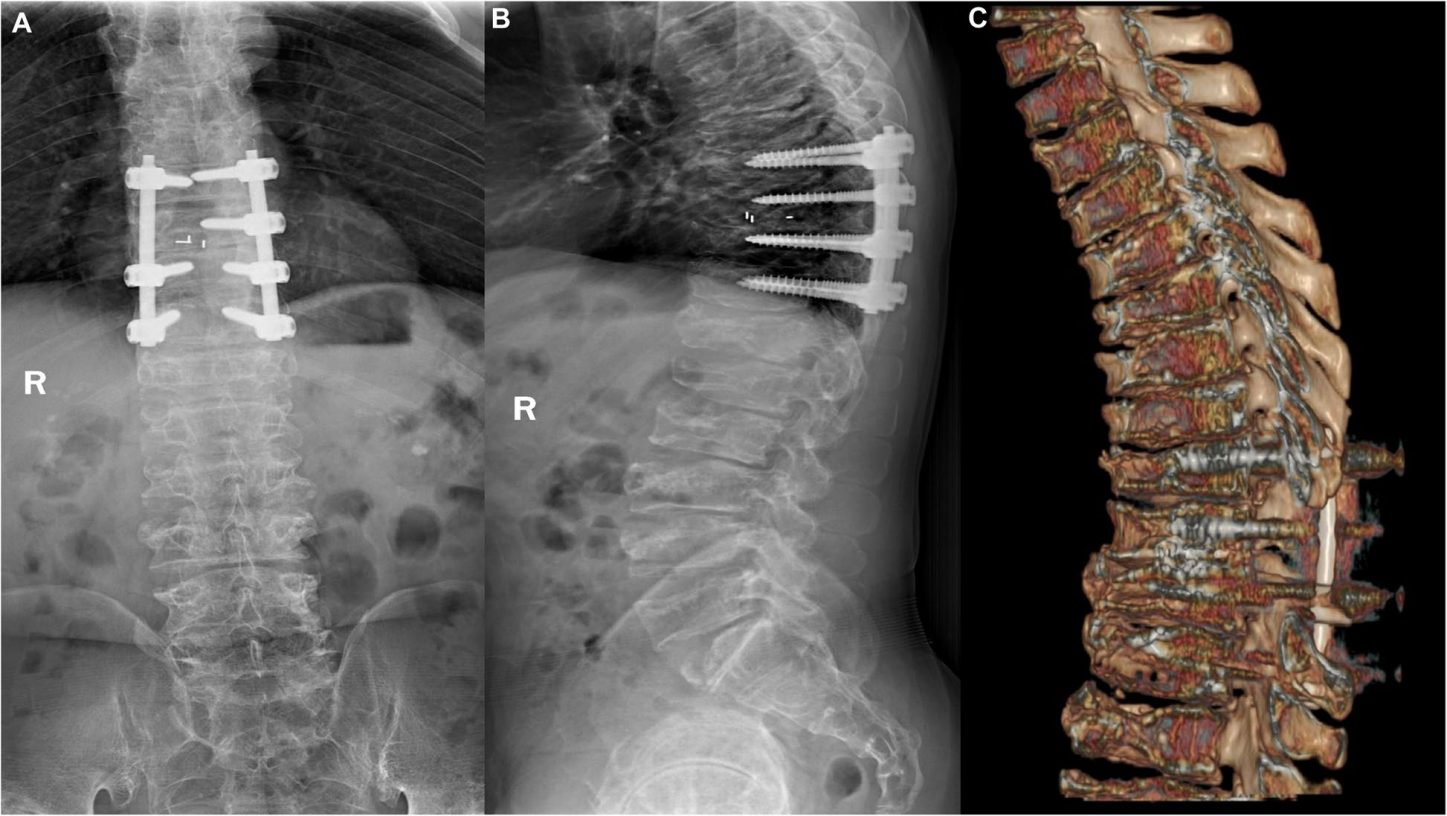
The one-year follow-up X-ray and three-dimensional CT (a, b, c) showed that T8–11 internal fixation was in place, T9–10 intervertebral high density shadow
